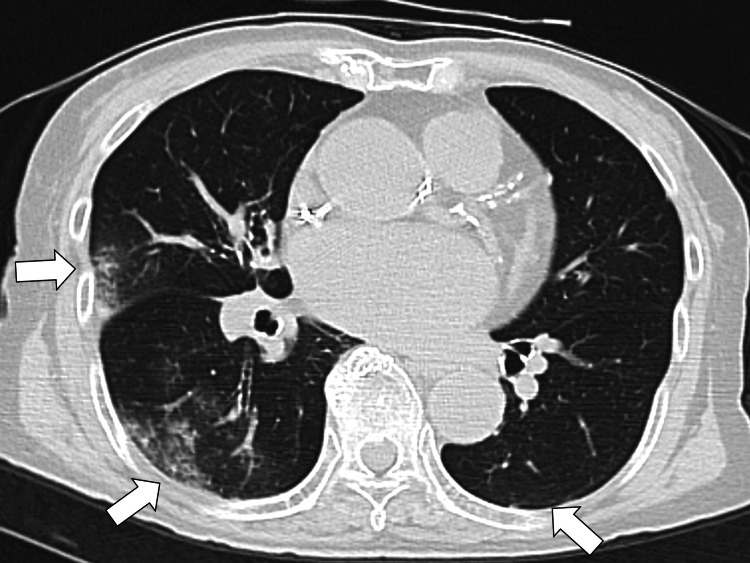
Chest computed tomography showing bilateral lung congestion (white arrows)
